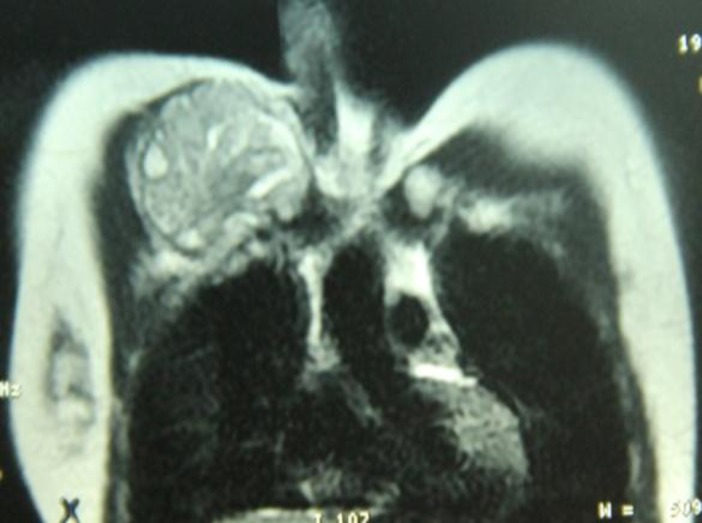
Coronal T2-weighted MRI shows a lobulated well-defined mass in right anterior chest wall arising from medial clavicle with bony destruction and upward extension to supraclavicular region consistent with chondrosarcoma.
